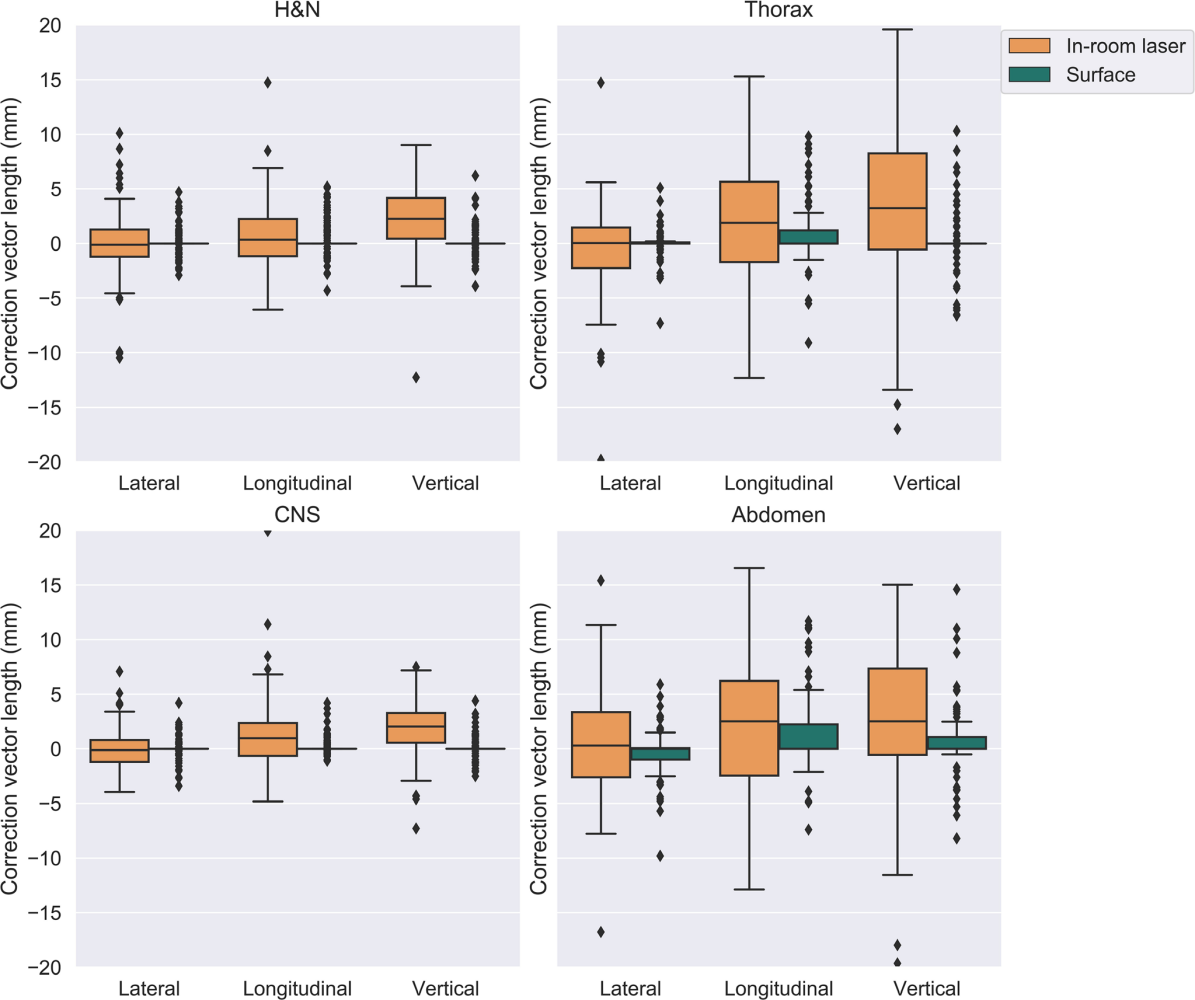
[Setup correction per axis and image modality] The residual error for surface scanning and the residual error for in‐room lasers, plotted per axis and treatment site. The residual error was assessed from the setup correction with megavoltage computed tomography to CT. Shown as a box‐and‐whisker plot, where the mid‐line represents the median (line), the interquartile range (box) and 1.5 times past the quartile range (outer line) and outliers (black point)
